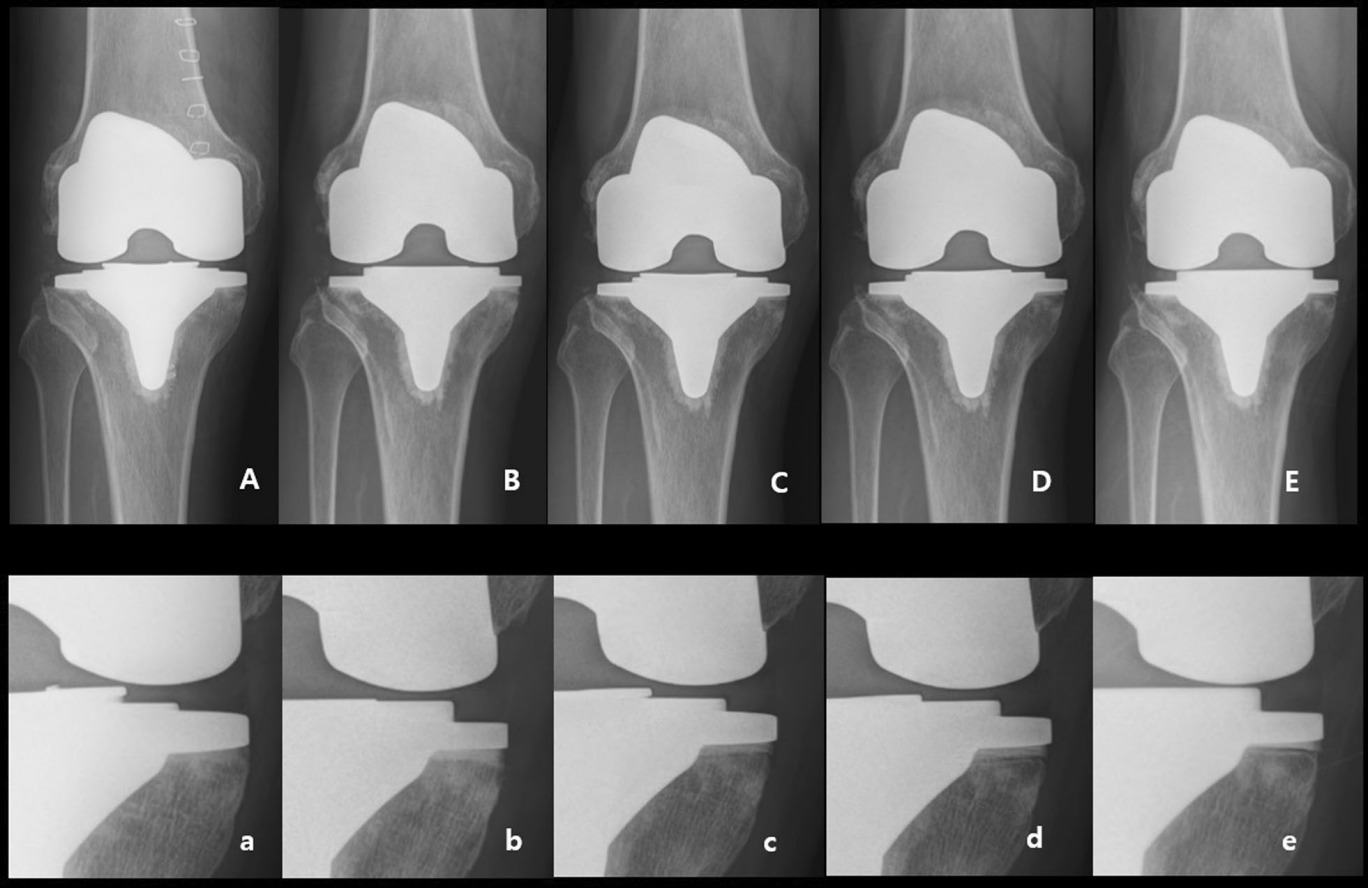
Serial anteroposterior radiographs of a 70-year-old female patient who underwent surgery using the Attune S +. Radiolucent line in cement–bone interface was visible at medial proximal tibia starting from the 6 months follow up after surgery. A, immediate postoperative; B, 6 weeks after surgery; C, 6 months after surgery; D, 1 year after surgery; E, 2 years after surgery
